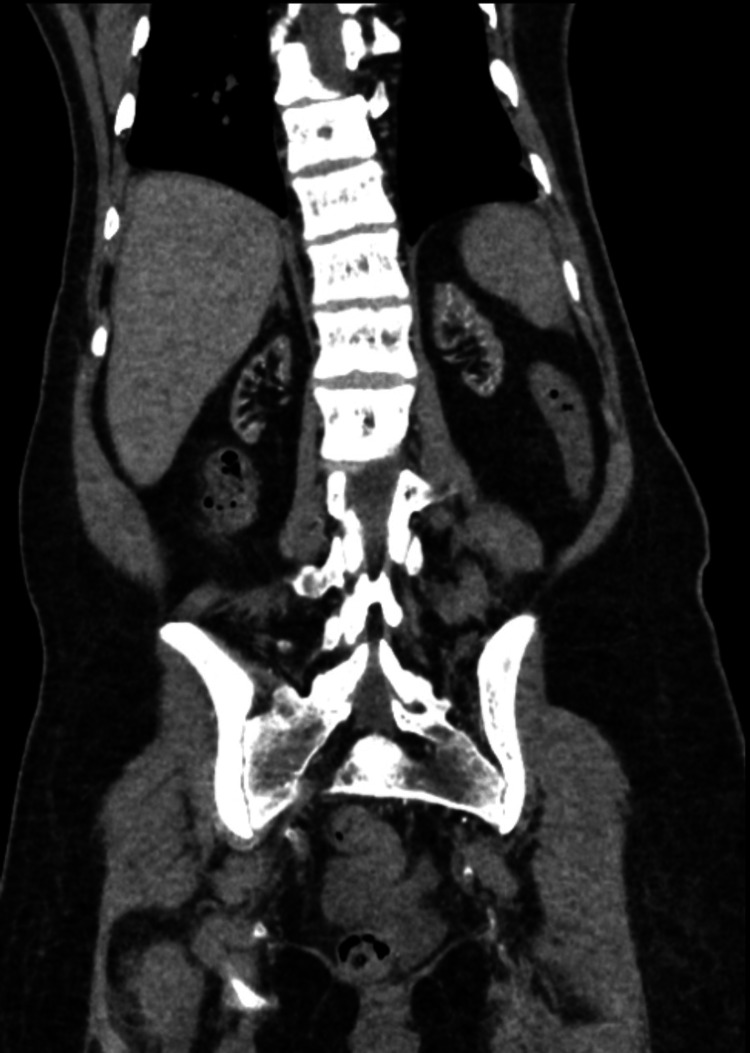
CT of the abdomen and pelvis demonstrating atrophic kidneys, consistent with the patient’s diagnosis of end-stage renal disease.
